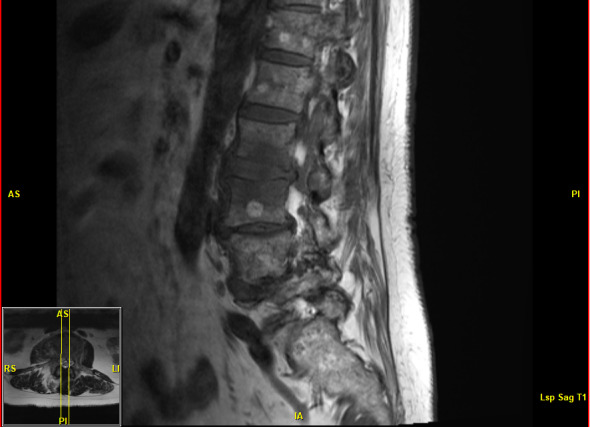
MRI demonstrating abnormal T2 hyperintensity with accompanying inflammatory changes at the L2–L3 level. Chronic multilevel
